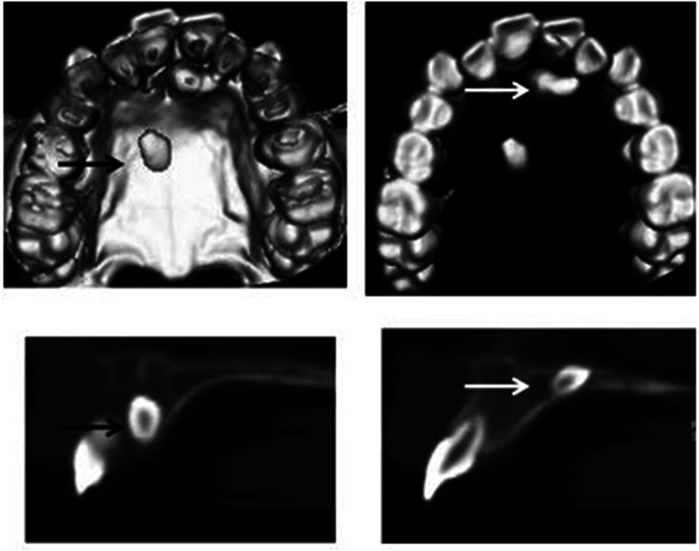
Cone beam computerized tomography 3D images are used for the localization of supernumerary teeth [arrows].
